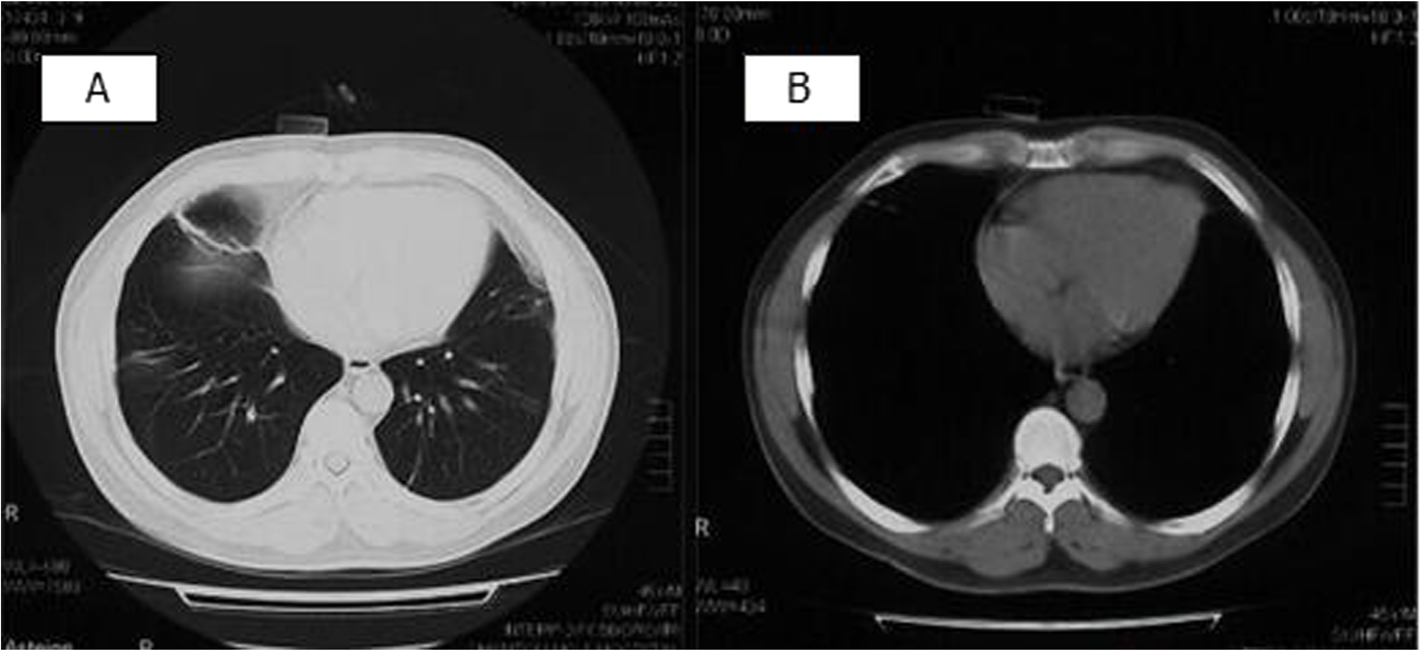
Follow-up chest CT scan of case 3. Total regression of PE, consolidation in the lower right lung and pericardial effusion with no recurrences
